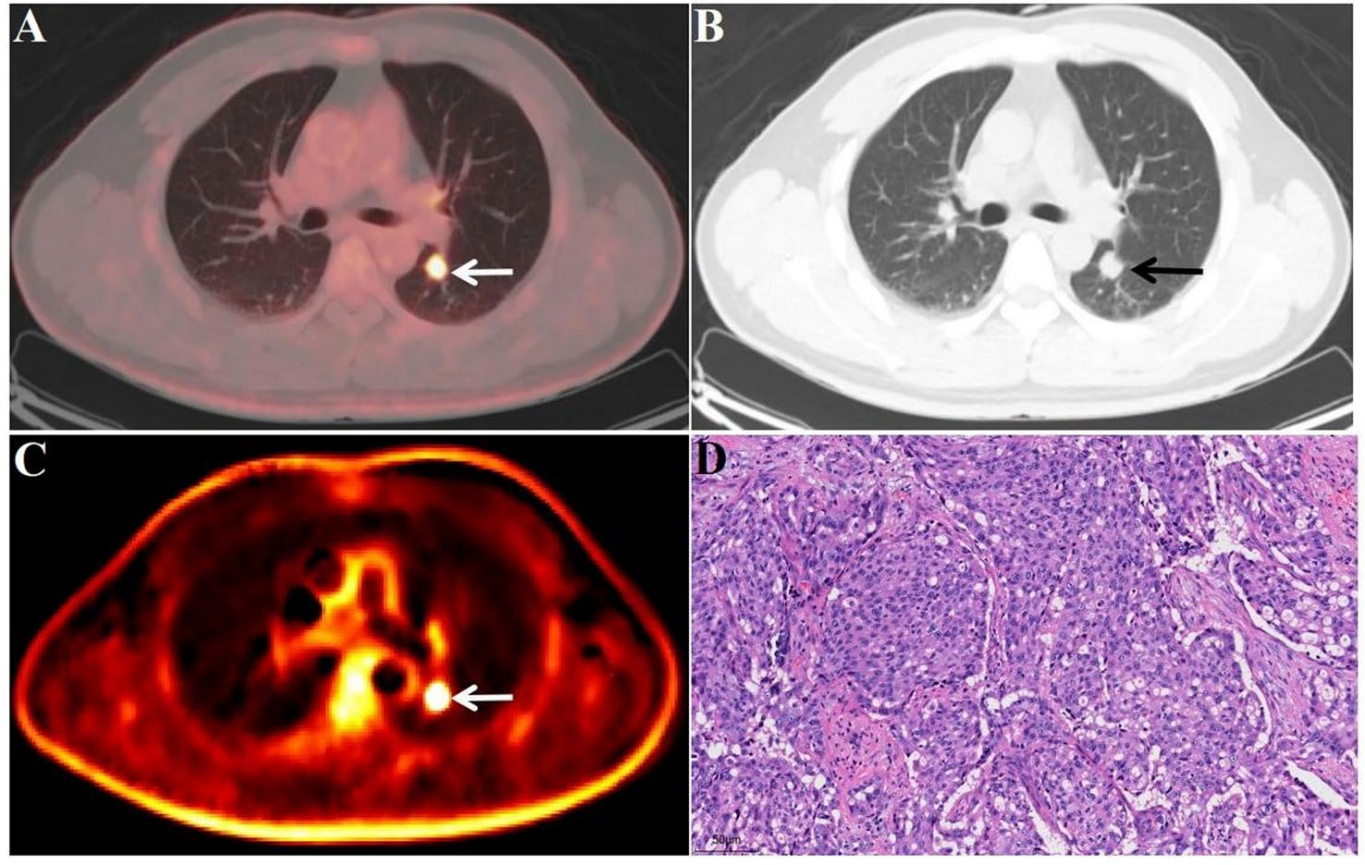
FDG-PET/CT images of a malignant lesion A 41-year-old male patient. Surgical pathology confirmed an adenocarcinoma (D, 20x field of view) in the upper lobe of the left lung (white/black arrow), with a size of 1.6 × 1.5 cm (A and B, white/black arrow), SUV_max_ of 13.8 (A, white arrow), and K_i_ of 0.0282 ml/g/min (C, white arrow). Postoperative EGFR test results showed EGFR exon 19 mutation
